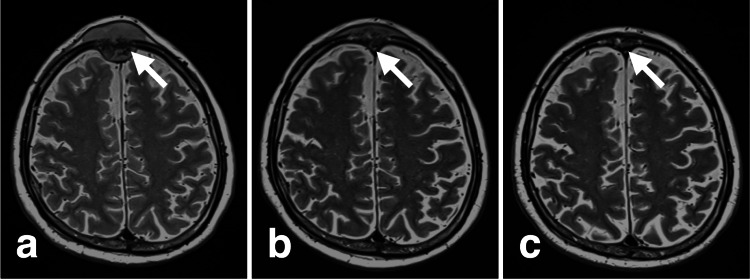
Time course of the frontal lesion on MRI T2WI (white arrows) (a) pre-CKR, (b) Day 5 after starting CKR, (c) Day 44 after starting CKR MRI: magnetic resonance imaging, T2WI: T2-weighted image, CKR: CyberKnife radiotherapy
